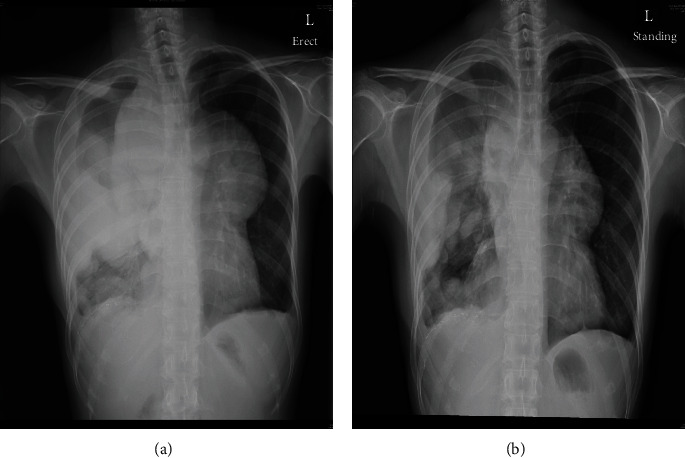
(a) Chest radiograph before thoracic radiotherapy. (b) Chest radiograph 4 months after thoracic radiotherapy.
